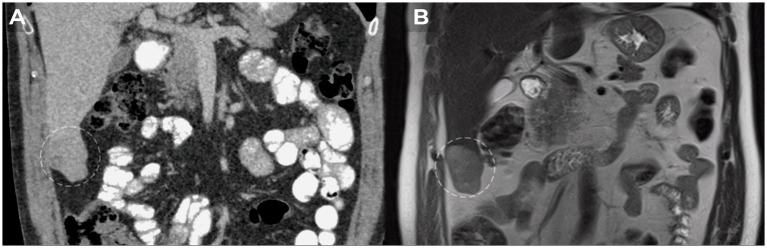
MRI dynamics of liver tumor growth. (A) Heterogeneous mass with clear irregular contours of 5×3 cm in the lower part of the 6th liver segment detected in 2021. (B) Growth dynamics of the liver tumor up to 7×5 cm in 2022.
